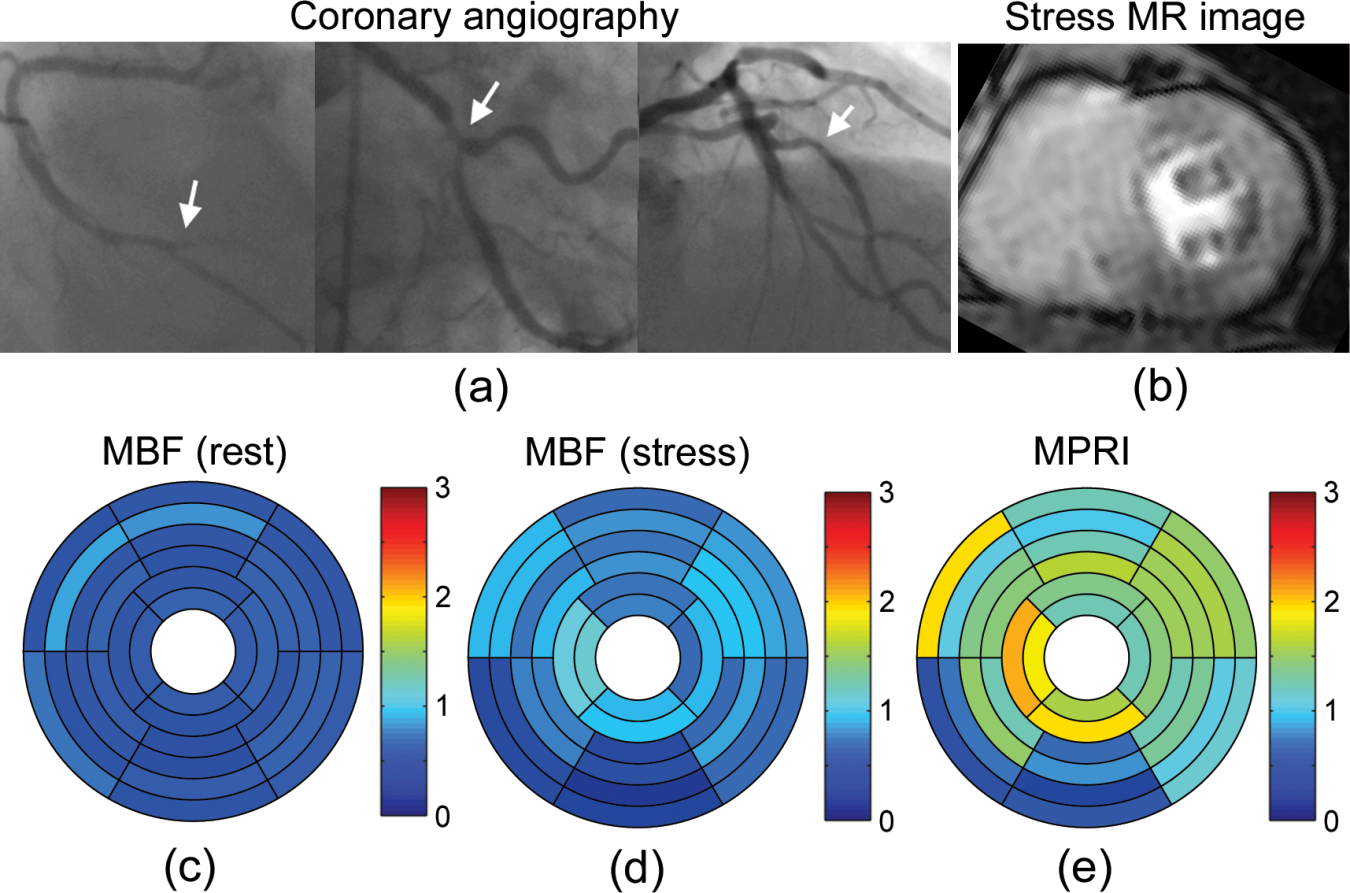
(a) Selective coronary angiography in a representative patient (66-year-old male) with severe triple-vessel disease (arrows). (b) First-pass enhancement mid-level MR image at stress showing a qualitatively delayed and decreased delivery of contrast agent to the inferior segments and milder delayed delivery to the anterior segments. Bullseye plots of MBF (ml/g/min) (c) at rest and (d) at stress, and (e) MPRI calculated from the NTH_*f*_ method.
